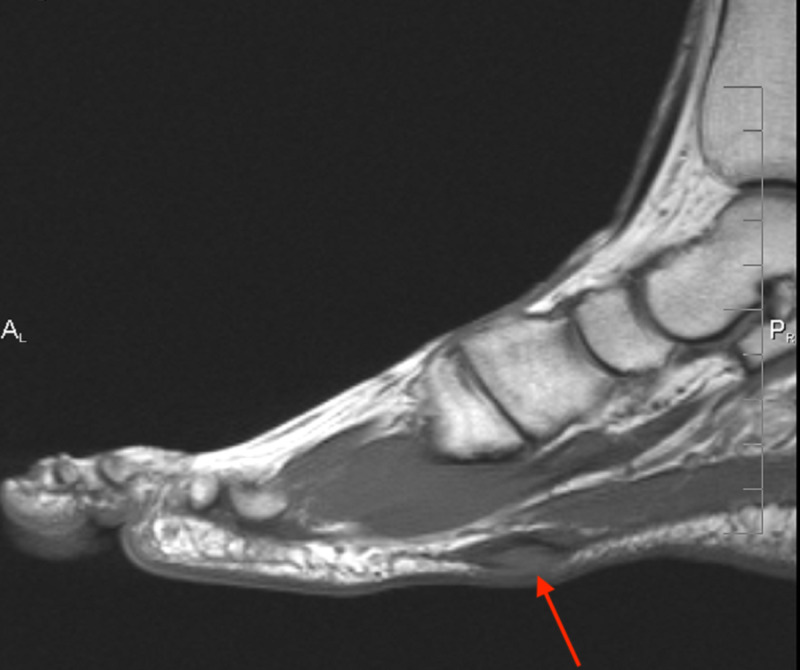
Case 1 MRI showing the sagittal T1 weighted image of the left foot demonstrating a plantar fibroma starting at the first tarsometatarsal joint with no involvement of the plantar musculature or flexor tendons.
